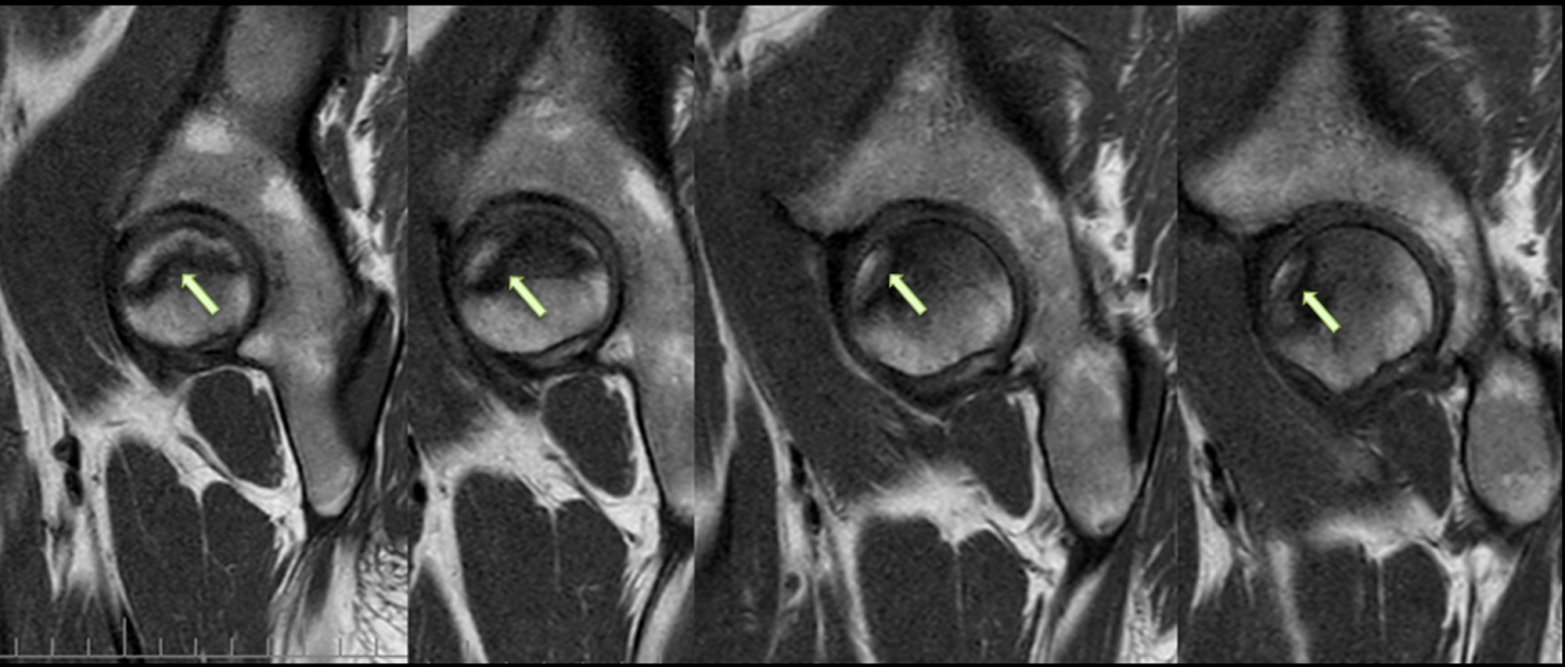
Sagittal T2-weighted MRI of the pelvis showing AVNFH AVNFH: avascular necrosis of the femoral head; MRI: magnetic resonance imaging
